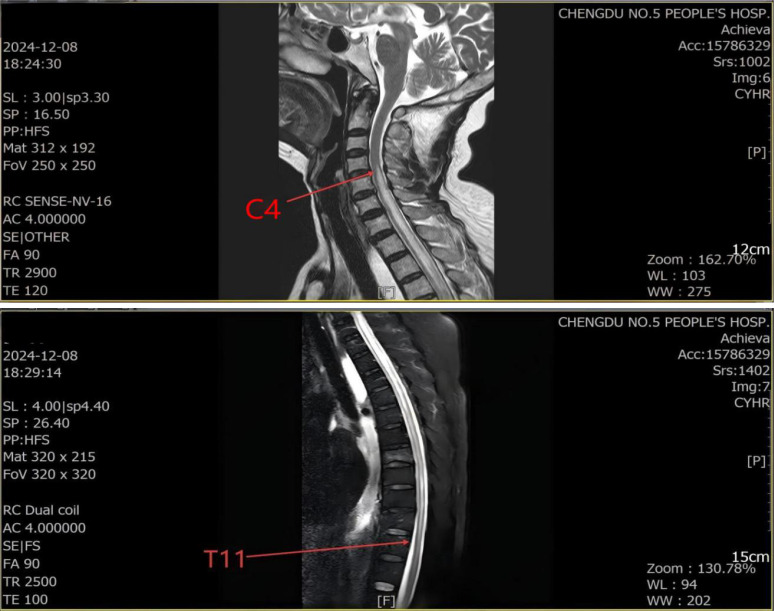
MRI report suggests: C4–T11 vertebral body plane spinal cord abnormal signal, the nature to be determined, considering the possibility of inflammatory lesions.
